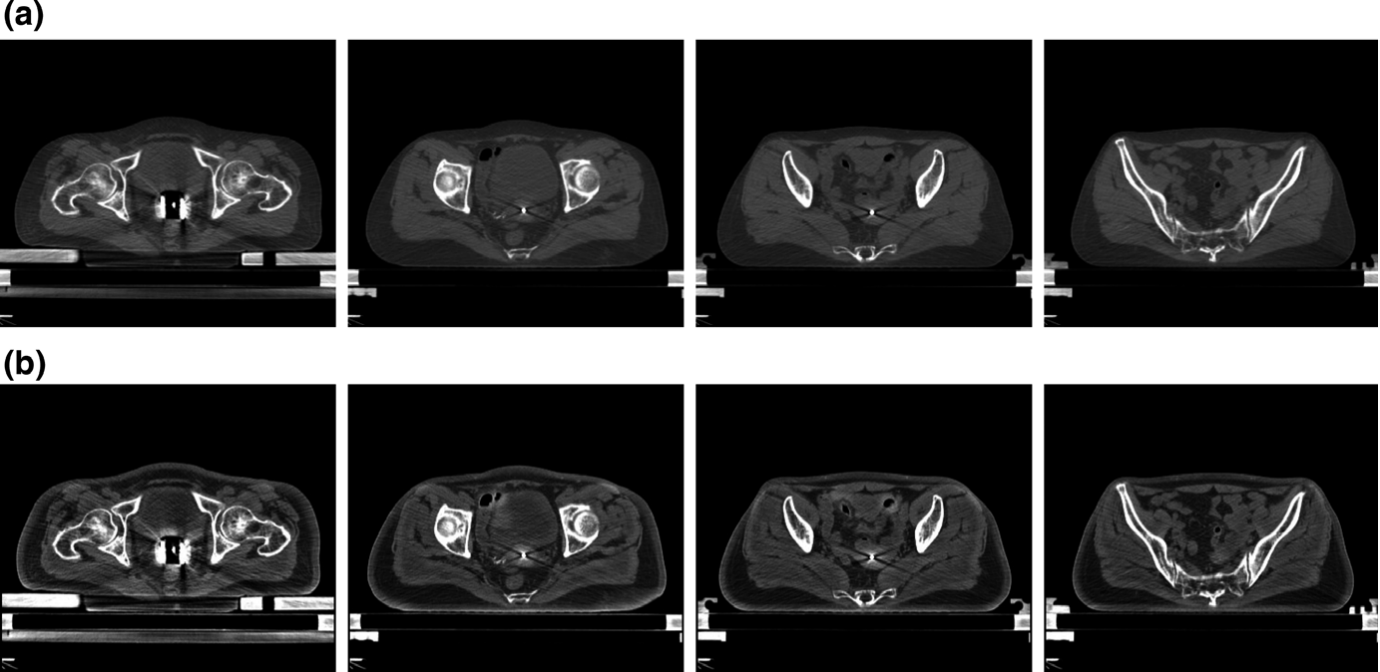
Computed tomography (CT) image enhancement using CLAHE algorithm. (a) Shows the original CT images. (b) Shows the enhanced CT images
